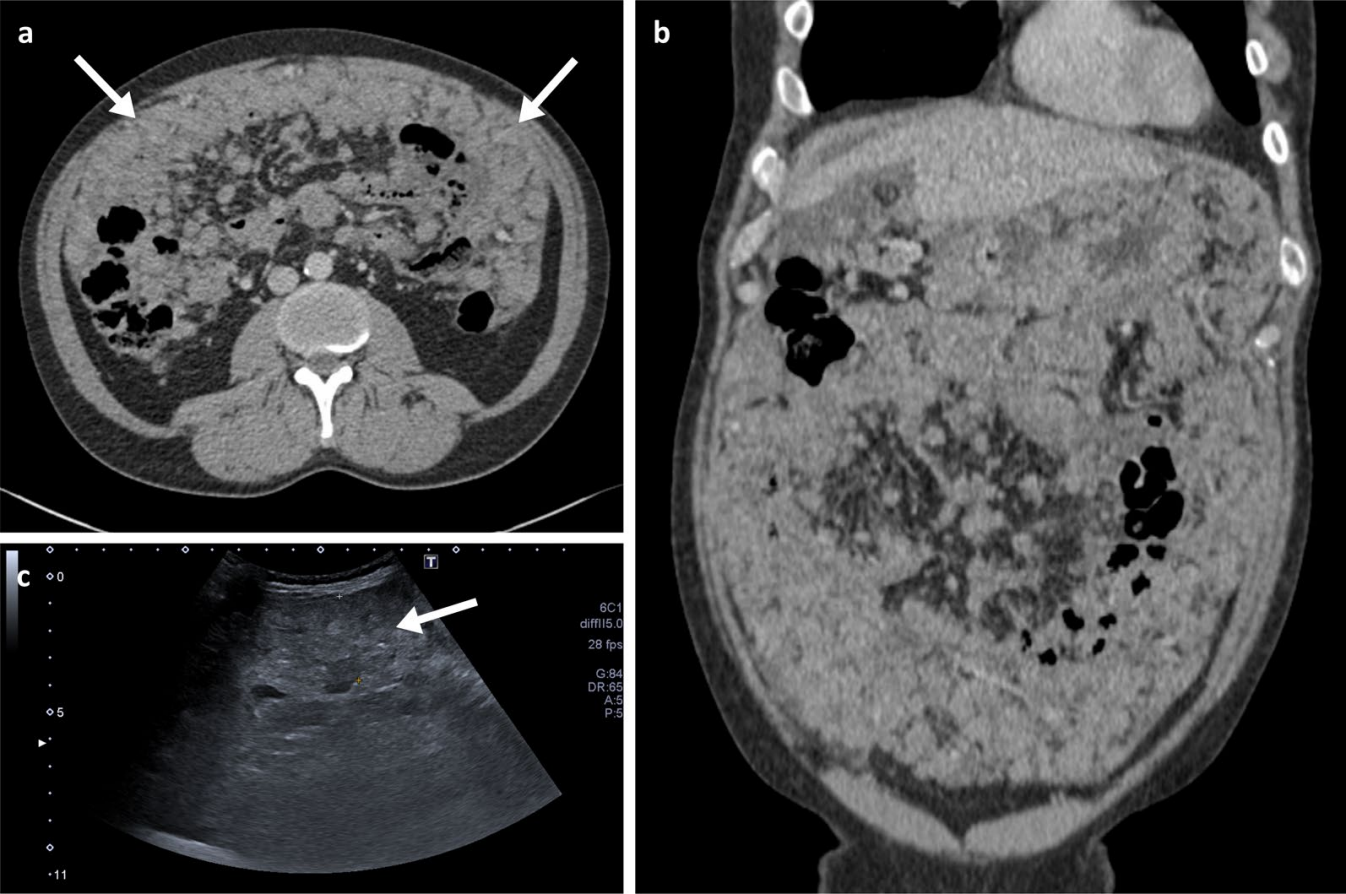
Portal phase contrast-enhanced CT axial (a) and coronal (b), and abdominal ultrasound (c) of a 48-year-old male patient with extensive metastatic melanoma peritoneal disease with innumerable nodules and omental cake (arrows)
